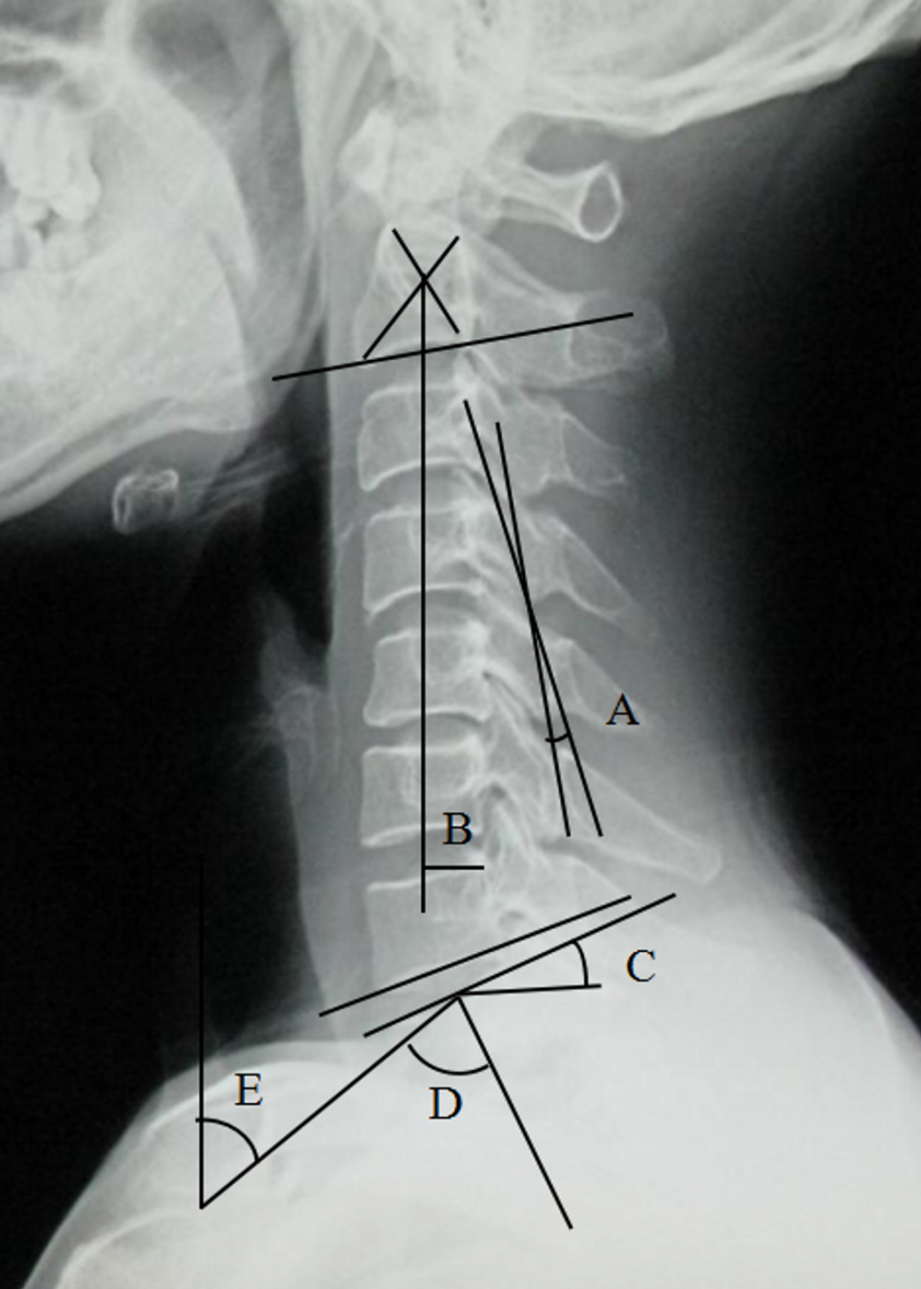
Preoperative radiographic measurement. (A) CL, (B) C2–7 SVA, (C) T1S, (D) TIA, (E) NT.
